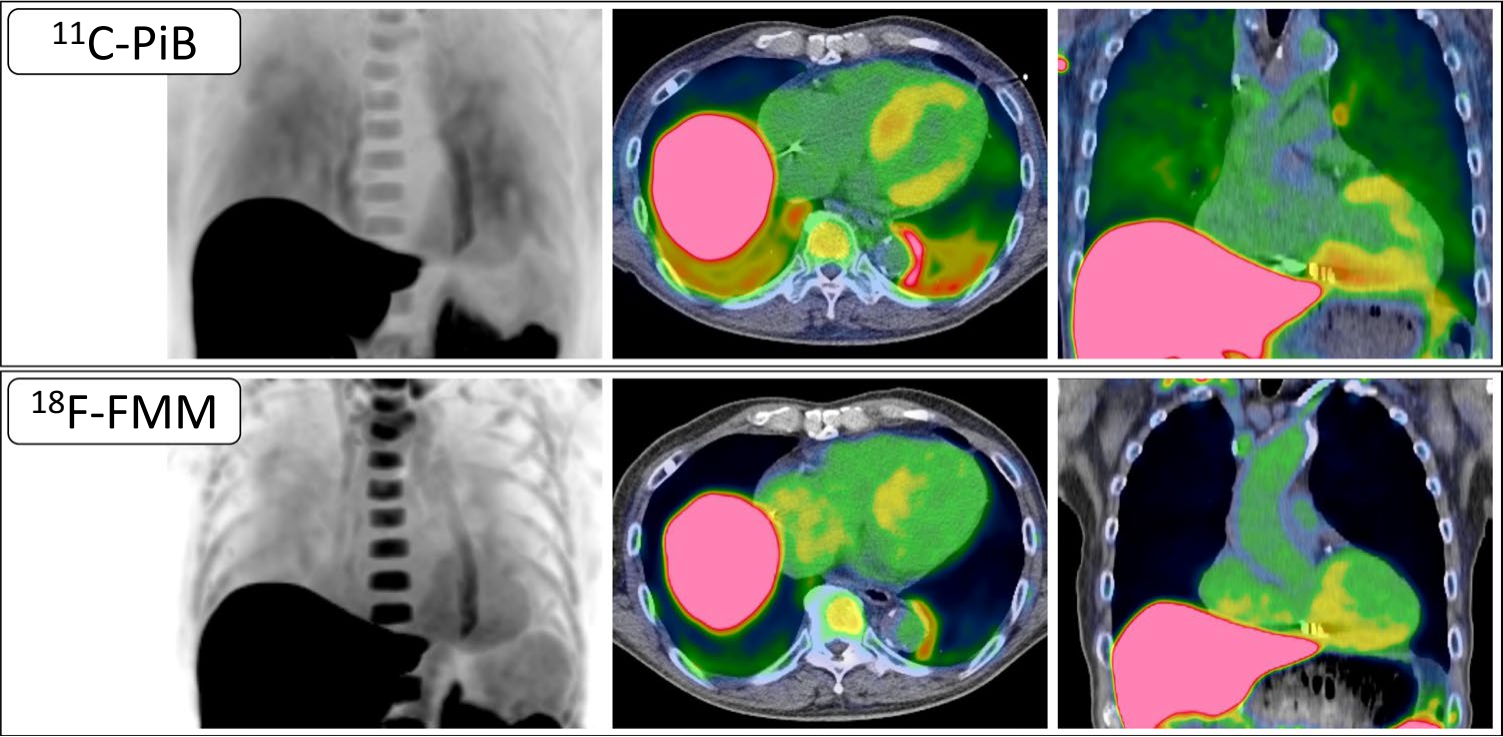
Representative PET imaging of transthyretin cardiac amyloidosis. Representative amyloid PET images from a male patient in his 80 s with wild-type transthyretin cardiac amyloidosis. The upper row shows images with ^11^C-Pittsburgh compound B (PiB), and the lower row shows images with ^18^F-florbetaben (FMM). From left to right: maximum intensity projection, axial PET/CT, and coronal PET/CT images. Both tracers demonstrate myocardial uptake predominantly in the basal segments of the left ventricular wall
